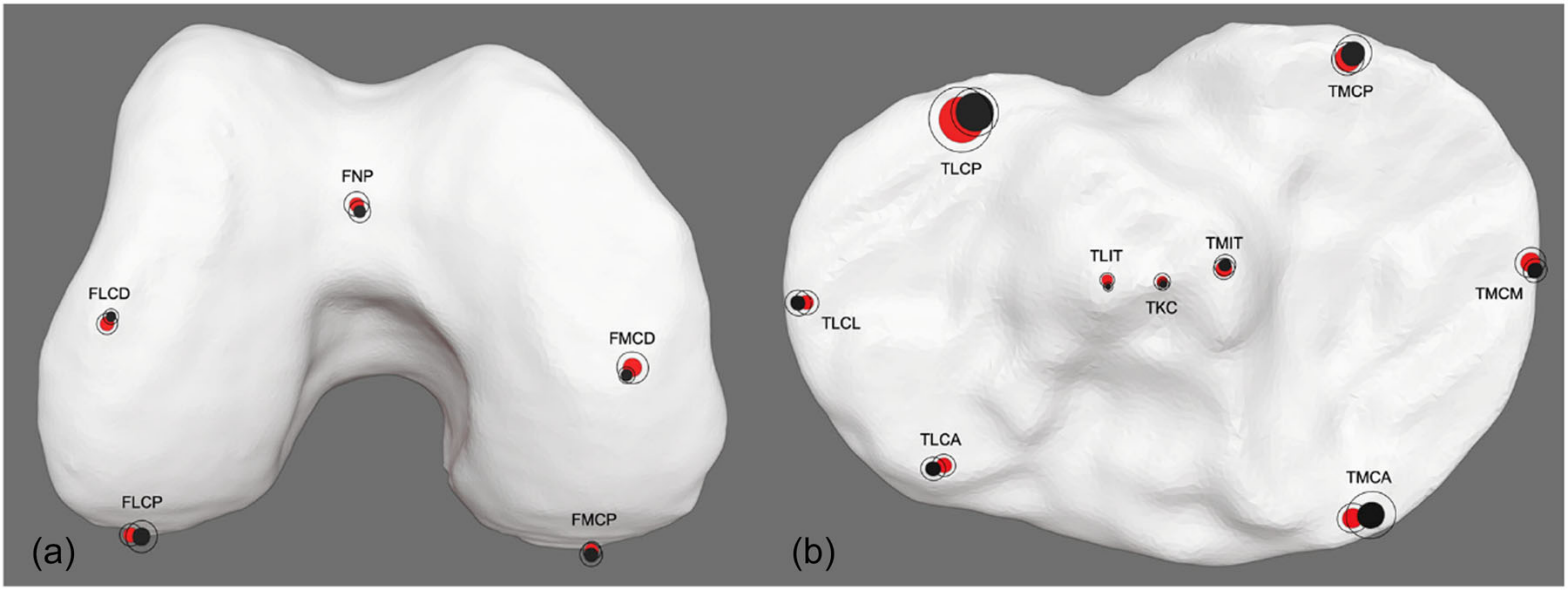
Bony landmarks of the distal femur (caudal to cranial view, a) and proximal tibia (cranial to caudal view, b) in a 3D reconstruction by CBCT. The filled areas represent the mean error (black for MSCT and red for CBCT), while the thin black circles indicate the mean error plus one standard deviation. CBCT, cone bean computed tomography; FLCD, femoral lateral condyle distal; FLCP, femoral lateral condyle posterior; FMCD, femoral medial condyle distal; FMCP, femoral medial condyle posterior; FNP, femoral notch point; MSCT, multislice computed tomography; TKC, tibial knee center; TLCA, tibial lateral condyle anterior; TLCL, tibial lateral condyle lateral; TLCP, tibial lateral condyle posterior; TLIT, tibial lateral intercondylar tubercle; TMIT, tibial medial intercondylar tubercle; TMCA, tibial medial condyle anterior; TMCM, tibial medial condyle medial; TMCP, tibial medial condyle posterior.
